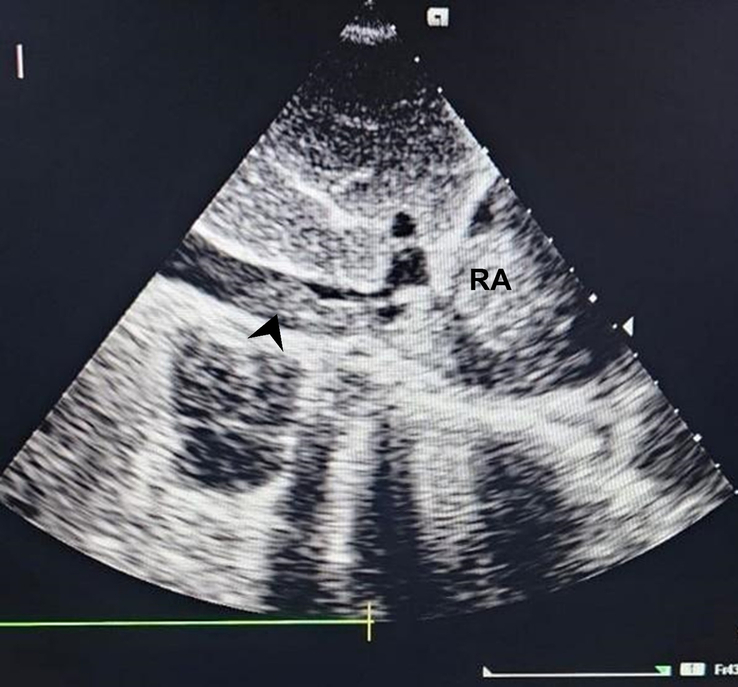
Transthoracic echocardiography—an echogenic mass protruding from the inferior vena cava (black arrowhead in the figure) into the right atrium (RA).
